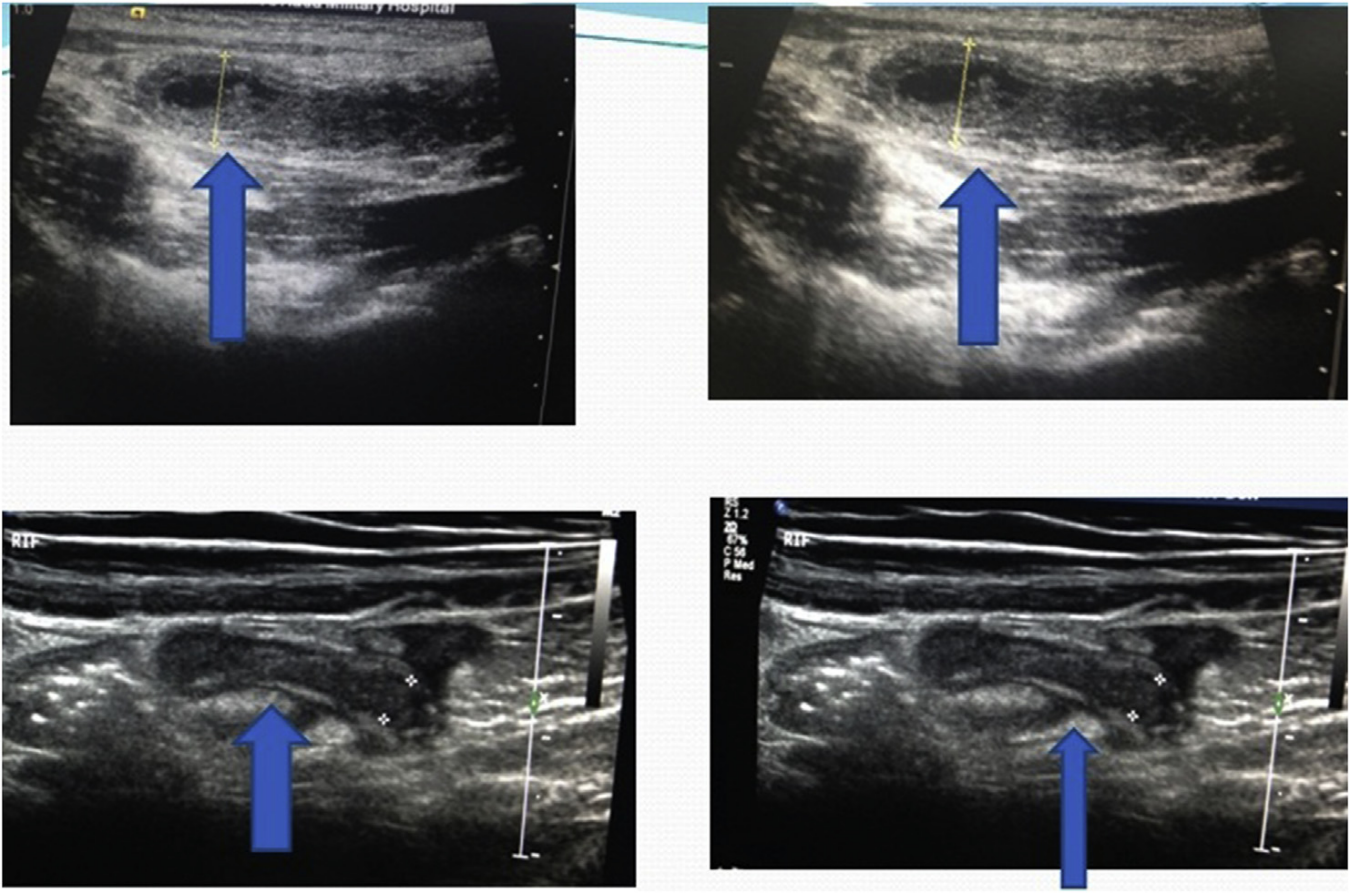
Ultrasonography images of appendix with arrow marks.
